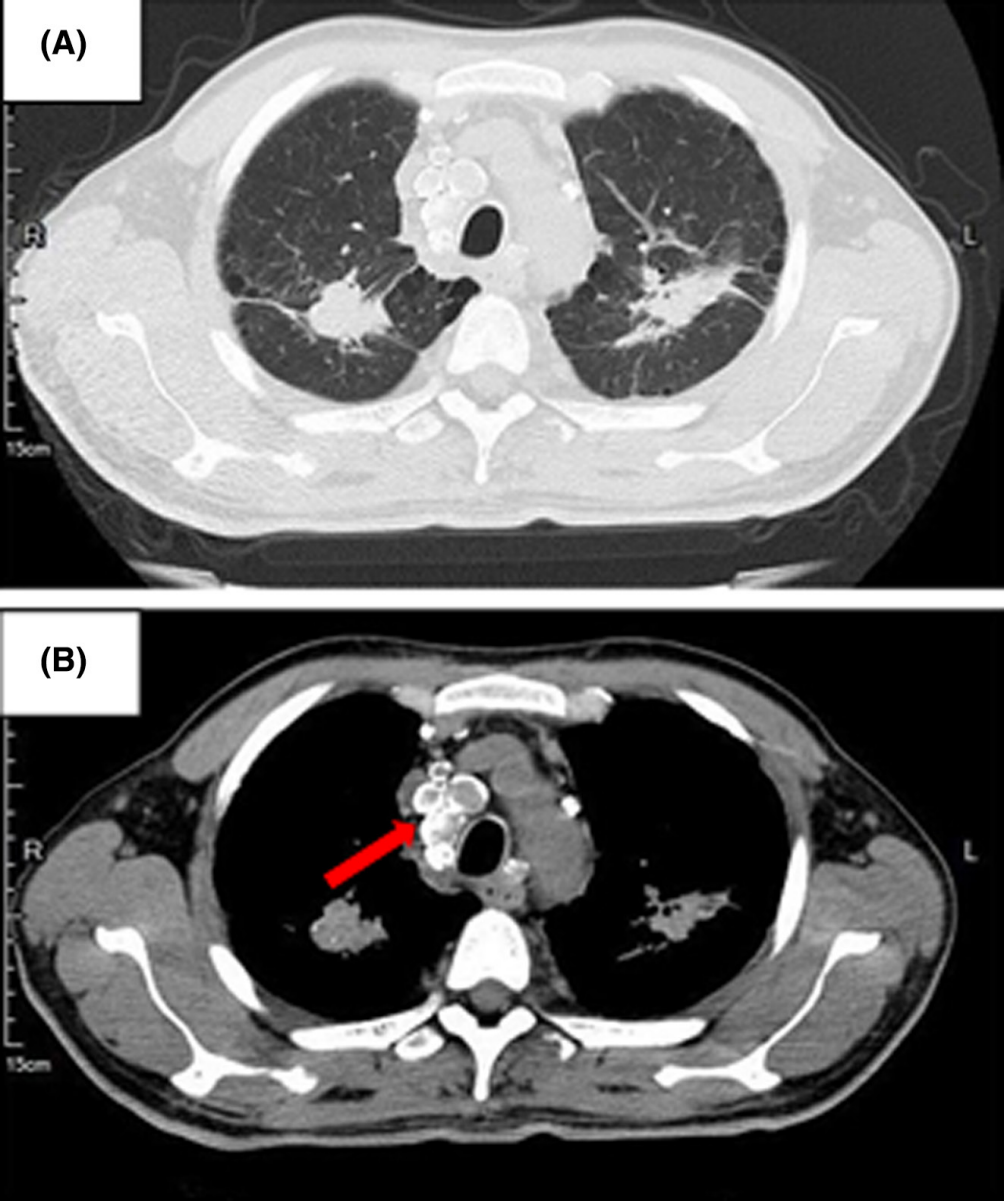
Chest CT imaging. (A) Multiple high‐density shadows of upper pulmonary lobe. (B) Red arrows indicate sites of multiple enlarged lymph nodes with calcification.
